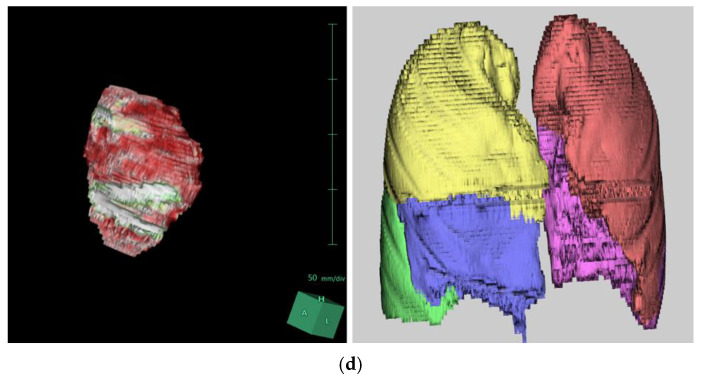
Three-dimensional reconstruction of the patient’s chest CT scan using SYNAPSE 3D: (a) Preoperative images. (b) Postoperative images following XI repair. (c) Three-dimensional reconstructed images of the heart and lungs before the repair. (d) Three-dimensional reconstructed images of the heart and lungs after the repair.
